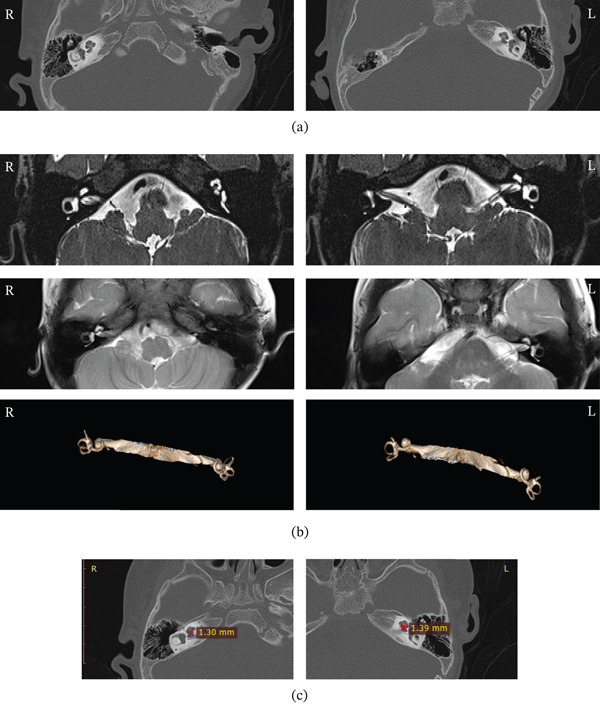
(a–b) Proband III: 1 temporal bone CT/MRI showed inner ear structures. Large vestibular aqueduct was excluded. (c) HRCT revealed cochlear aperture stenosis bilaterally with asymmetric severity (the final cochlear aperture width was calculated based on the average measurements obtained by the two reviewers and defined as cochlear aperture < 1.4 mm).
